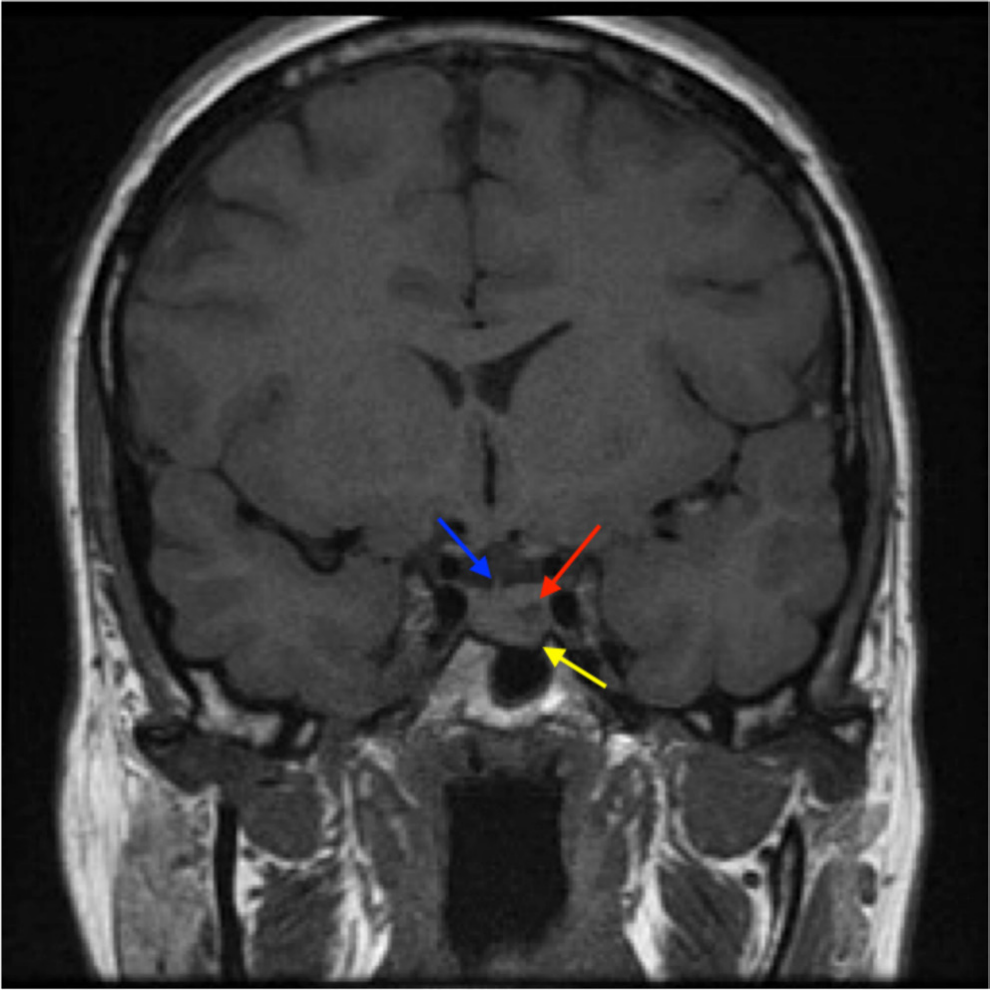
MR Scan in January 2010. Coronal T1-weighted MR scan revealing a mass within the pituitary gland on the left (red arrow). The pituitary stalk is deviated to the right (blue arrow) as a result and the floor of the pituitary gland is sloping down to the left hand side (yellow arrow) eroding into the pituitary fossa. These findings are entirely consistent with a pituitary microadenoma.
